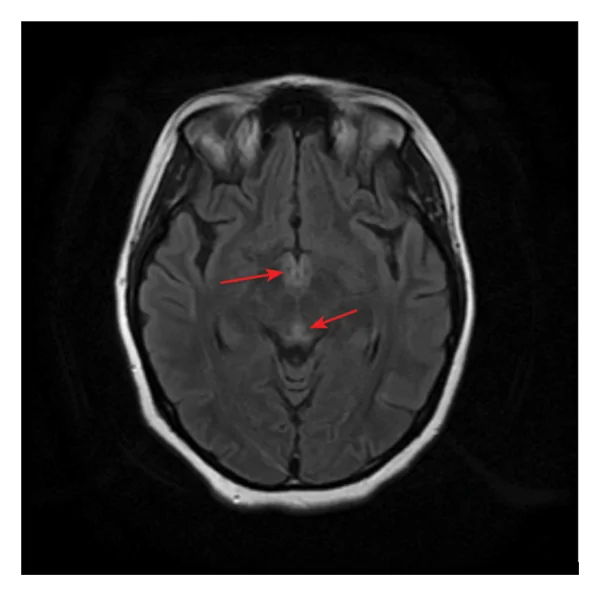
Symmetrical T2/FLAIR hyperintensities involving the bilateral medial thalami, periaqueductal gray matter, and mammillary bodies are demonstrated.
